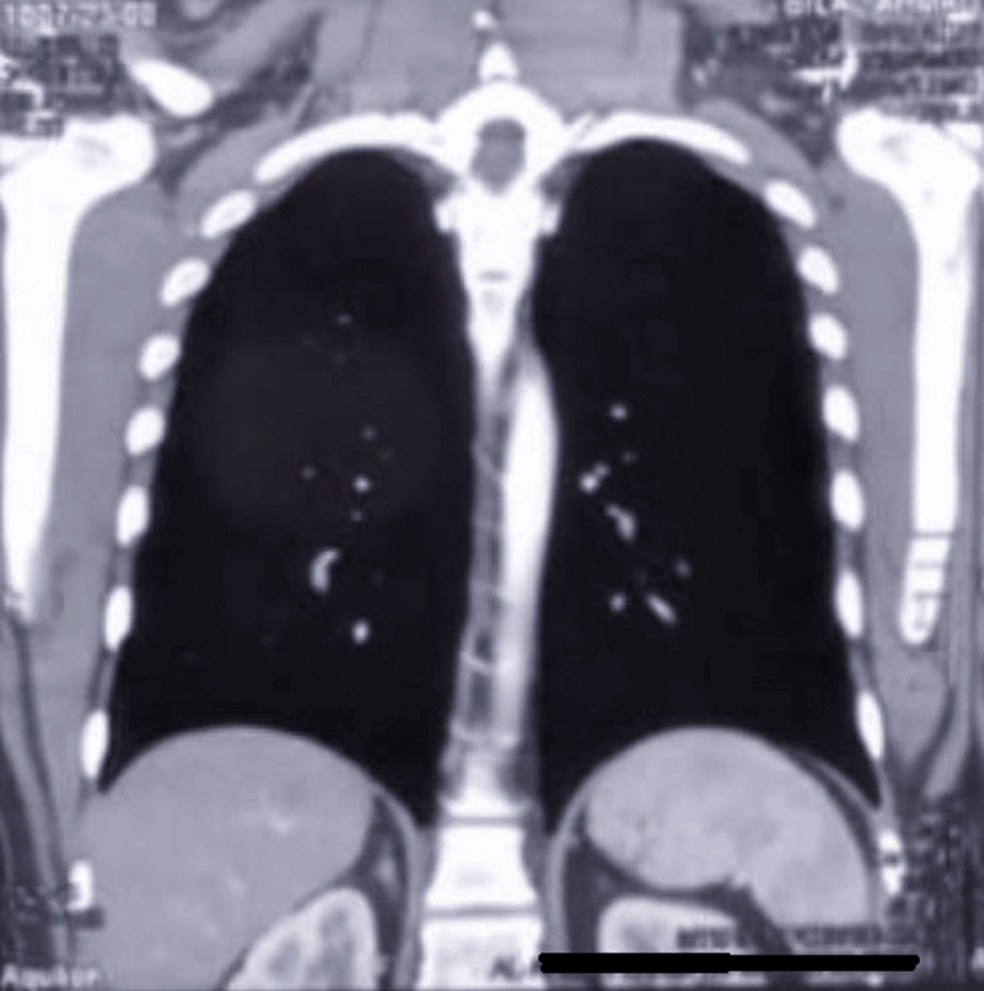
The CT scan of the chest showed no abnormalities and was deemed unremarkable
